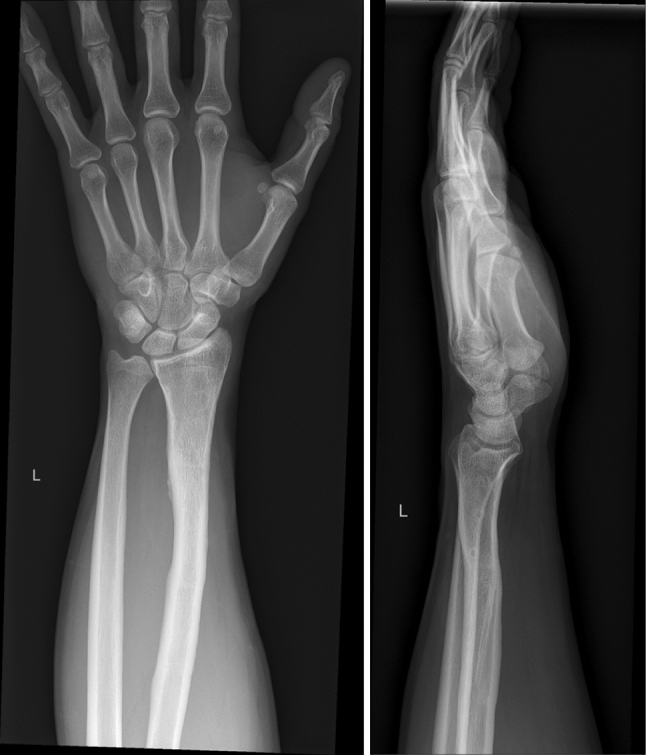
Conventional X-rays at 27 years dp and lat
